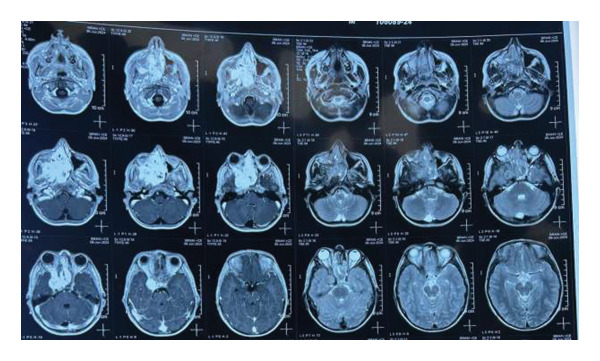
MRI brain with contrast axial view demonstrates a large lobulated, avidly enhancing mass lesion in the right nasal cavity and nasopharynx, with soft tissue signal intensity. On T2‐weighted images, the lesion appears intermediate with prominent flow voids and a few cystic areas, while on T1‐weighted images, it is isointense to muscle and does not show any diffusion restriction. The mass extends superiorly into the right sphenoid sinus, abutting the sellar floor, and anterosuperiorly into the right ethmoid sinus. Anteroinferiorly, it bulges into the right maxillary sinus and extends anteriorly into the orbital apex through the inferior orbital fissure, involving the greater wing of the right sphenoid bone and producing an anterior bulge.
